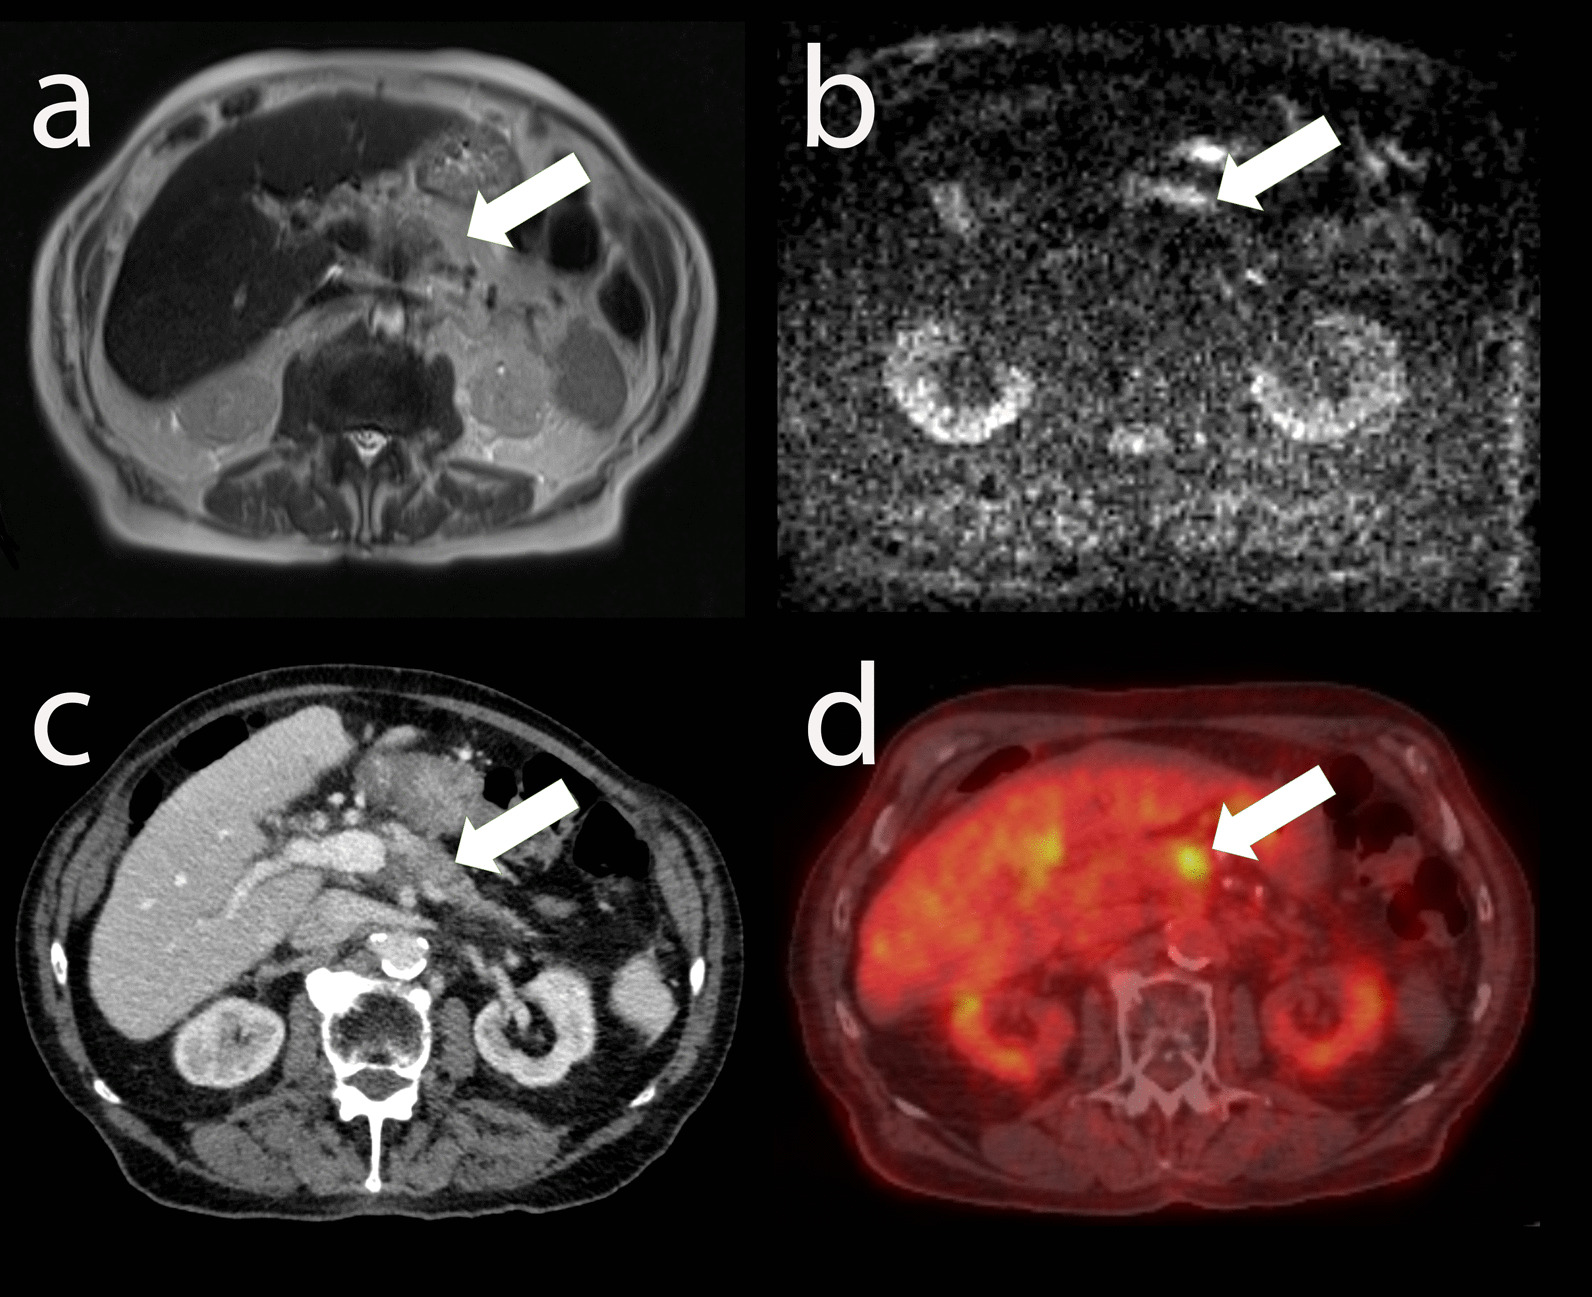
Axial T2 (a) and high *b*-value diffusion-weighted imaging (b) showing a lesion in the pancreatic body (arrows) prior to irreversible electroporation. Axial CT (c) showing a hypoenhancing soft tissue lesion (arrow) in the body of the pancreas that encases the mesenteric root vasculature and corresponds to a fluorodeoxyglucose (FDG)-avid lesion (arrow) on PET/CT (d)
